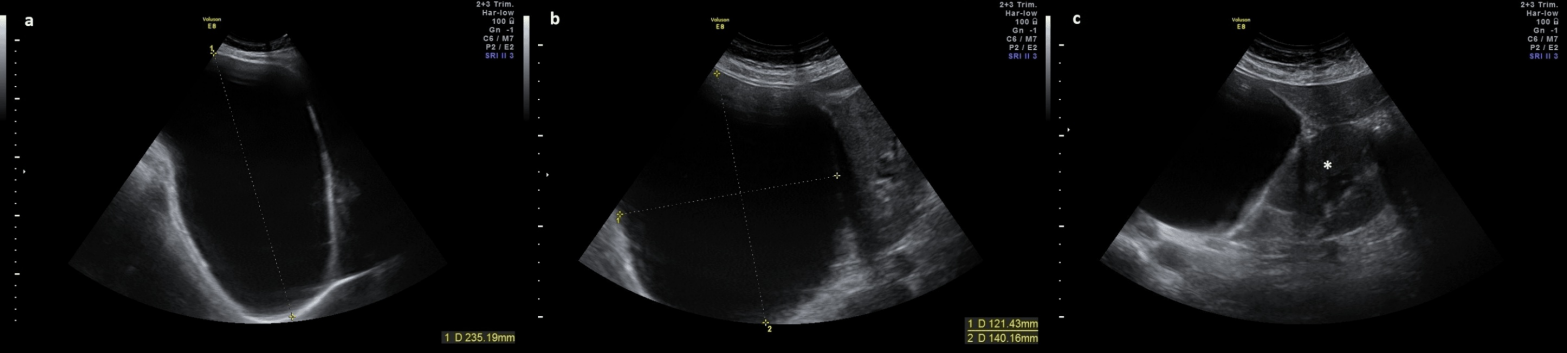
Antenatal abdominal US at 36 weeks of gestation showing a large, elongated anechoic retrohepatic cystic lesion with regular contours, measuring 235 × 140 × 121 mm, causing inferior and medial deviation of the right kidney. No Doppler flow was observed. Due to the deep retroperitoneal location, the uterus and the lesion cannot be visualized in the same image. (a) Coronal section. (b) Axial section. (c) Axial section showing the cystic lesion and right kidney (*). US: ultrasound
